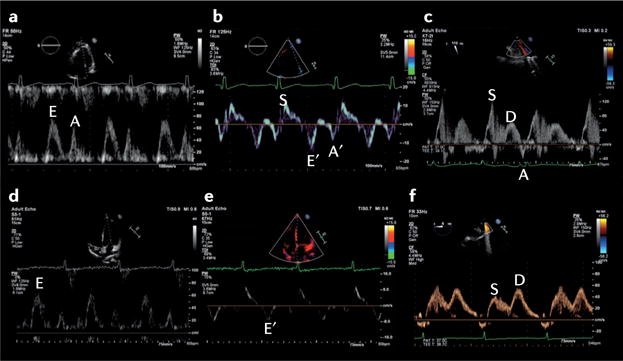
Echocardiographic methods to estimate left atrial pressure The upper panels show the echocardiographic scan of a patient aged 45 years admitted to hospital with dyspnoea owing to severe acute respiratory failure. a | Transthoracic echocardiogram (TTE) of the mitral inflow pattern showing a normal early (E) and late (A) transmitral flow pattern. b | Tissue Doppler imaging (TDI) of the lateral mitral valve annulus from the same patient; S is systolic annular velocity, E′ is early annular diastolic velocity, and A′ is late annular diastolic velocity (related to atrial contraction). c | Pulmonary venous Doppler (transesophageal echocardiography) demonstrating a dominant systolic wave (S) and smaller diastolic wave (D), with a normal deceleration time. The E/A ratio is >1 and the E/E′ is <8 cm/s with a dominant S wave on pulmonary vein, consistent with a normal left atrial pressure. The lower panels show the echocardiographic scan of a female patient aged 59 years admitted with dyspnoea owing to severe left ventricular dysfunction with pulmonary oedema. d | TTE of the mitral inflow pattern showing a dominant E wave with E/A ratio >2. e | TDI of the septal mitral valve annulus with a very low early diastolic velocity (E′), and f | pulmonary venous Doppler (transoesophageal echocardiography) showing a blunted systolic wave (S) and dominant diastolic wave (D). The E/E′ is 16.3 cm/s, and dominant D wave on pulmonary venous Doppler with D deceleration time <150 ms are consistent with an elevated left atrial pressure.
